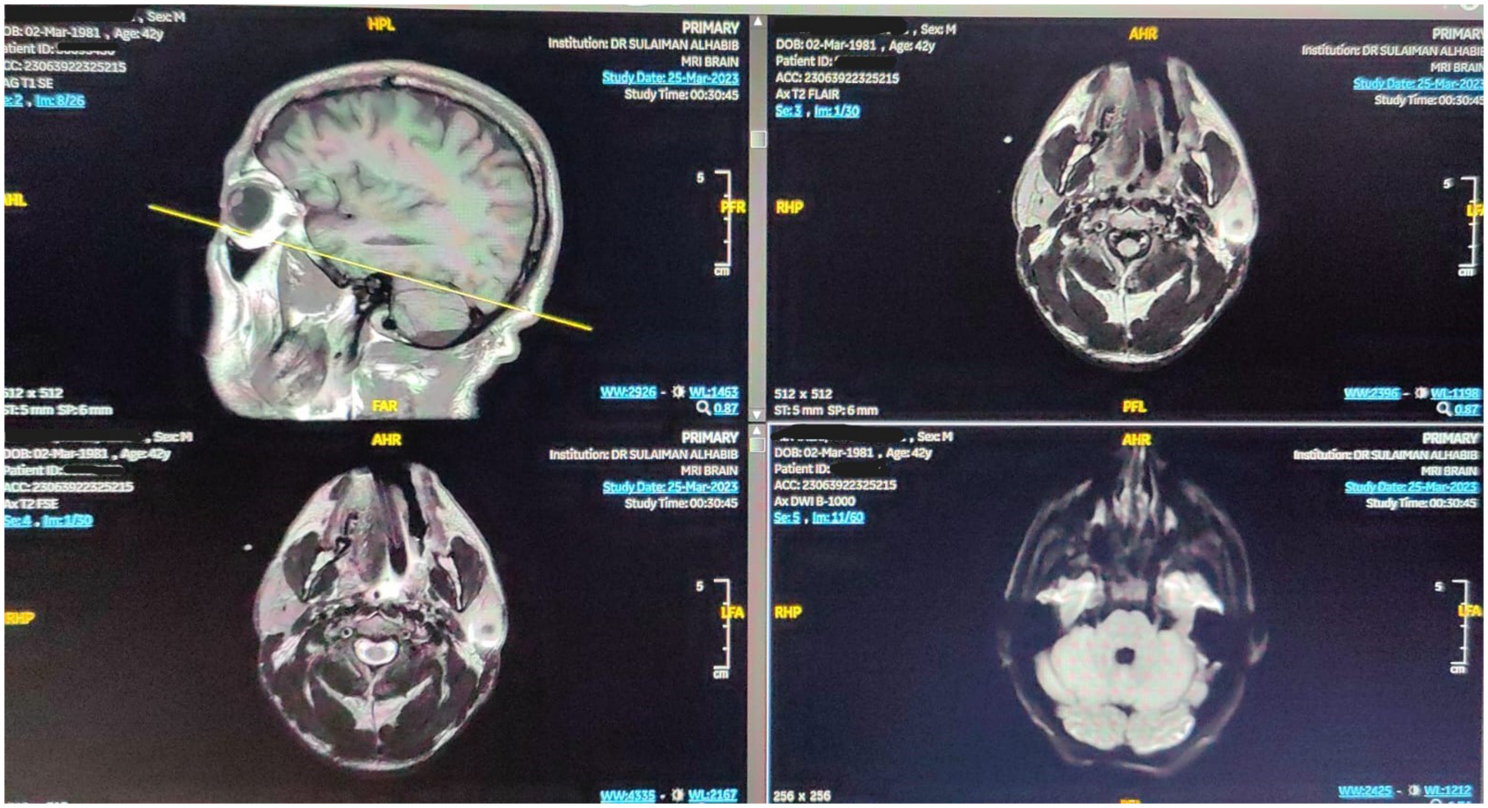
Non-contrast brain MRI. DWI shows cortical diffusion restriction predominantly in the parietal and parieto-occipital regions with corresponding low ADC; FLAIR shows cortical/subcortical hyperintensity.
